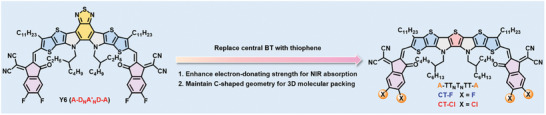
The chemical structures of Y6 and the C‐shaped A‐TT_N_T_N_TT‐A‐type electron acceptors, CT‐F and CT‐Cl, used in this research.
